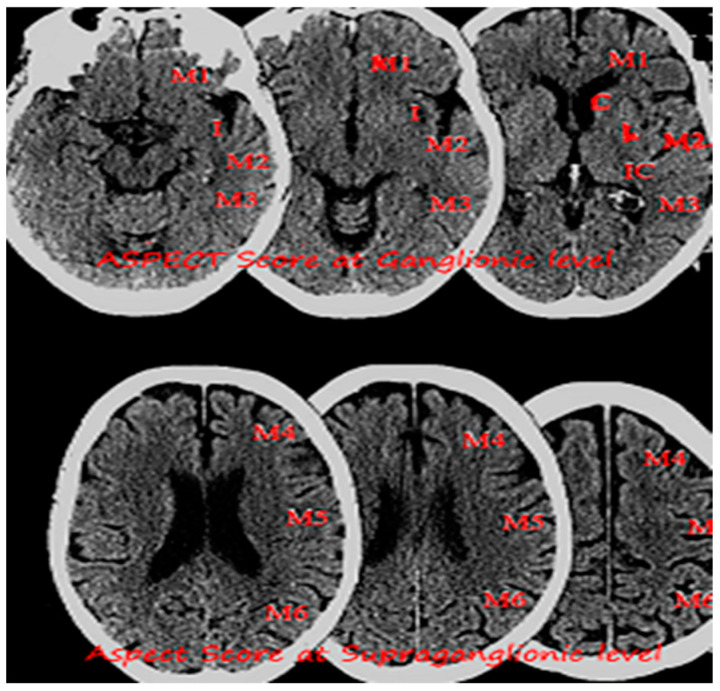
Image showing non-enhanced CT head with an Alberta Stroke Program Early CT Score (ASPECTS) at Ganglionic and Supra ganglionic level.
